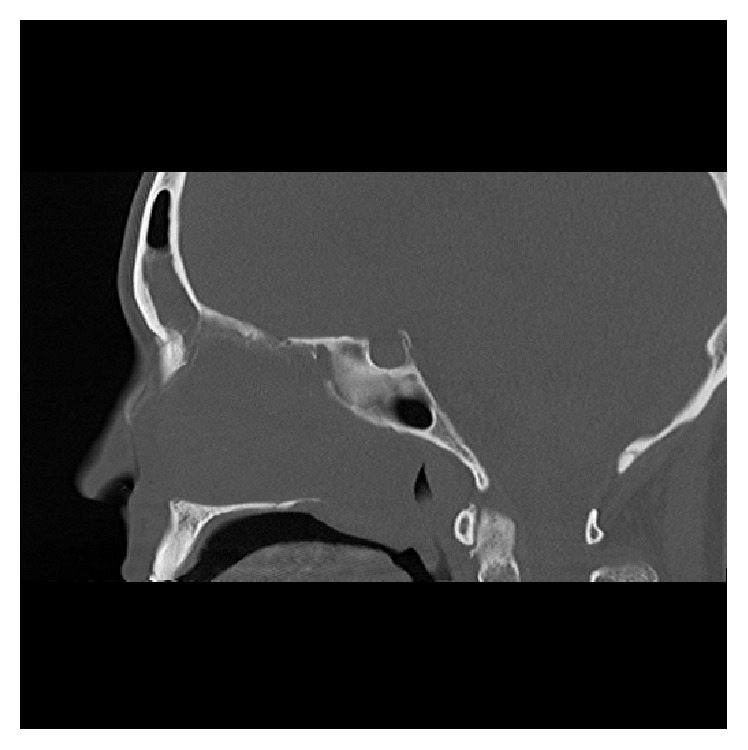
CT (sagittal view bone window).
